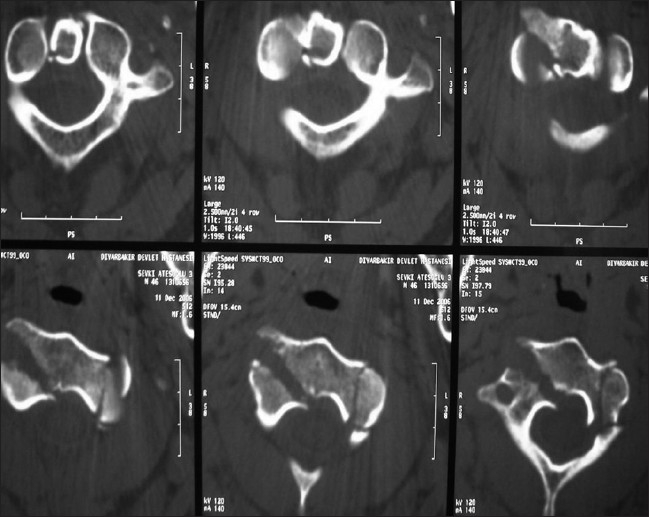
CT scan showed fracture of left pars interarticularis involving body of C2
